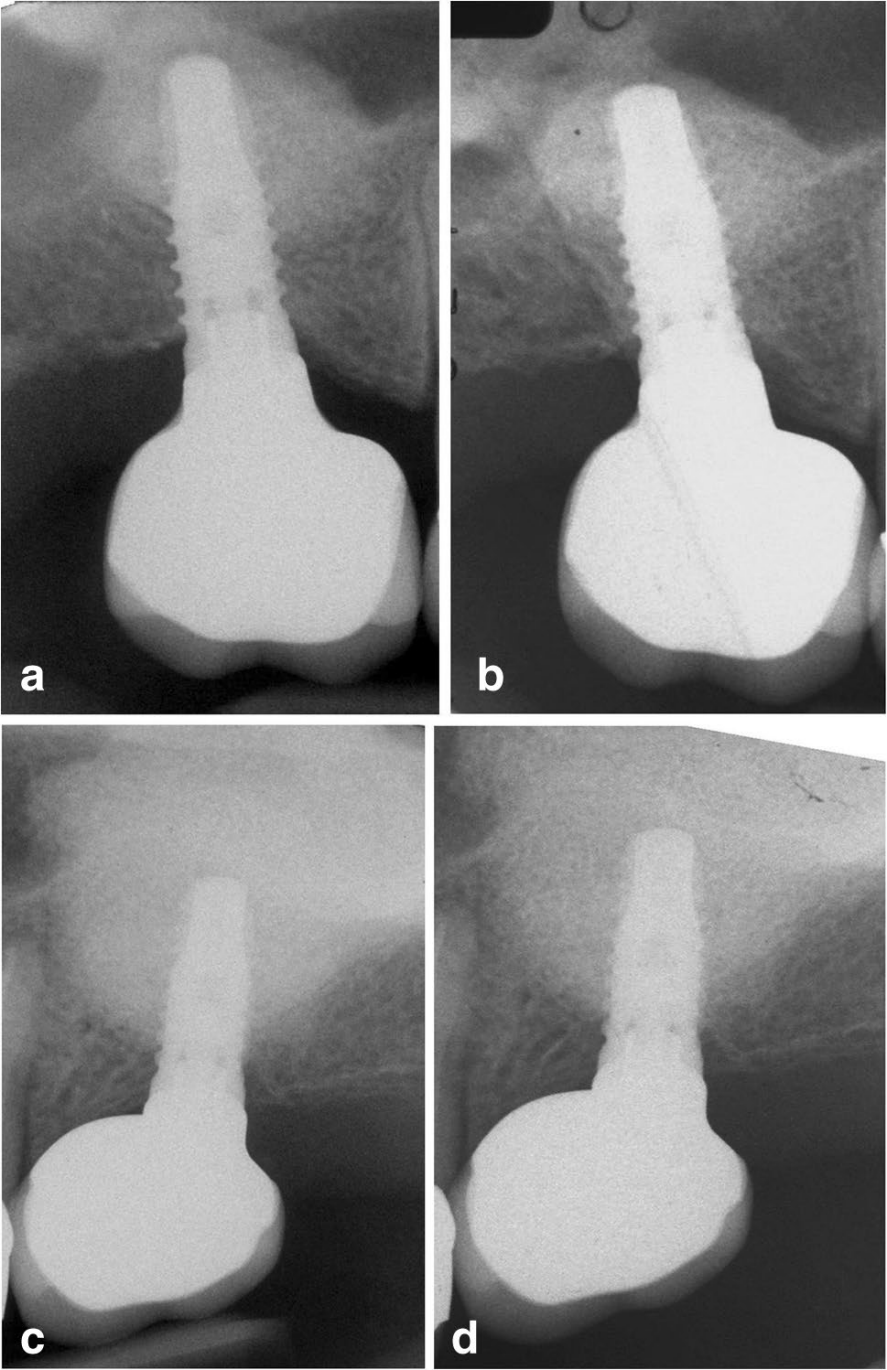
One- and 3-year follow-up periapical radiographs of two representative tSFE and lSFE cases. a tSFE, 1 year. b tSFE, 3 years. c lSFE, 1 year. d lSFE, 3 years
